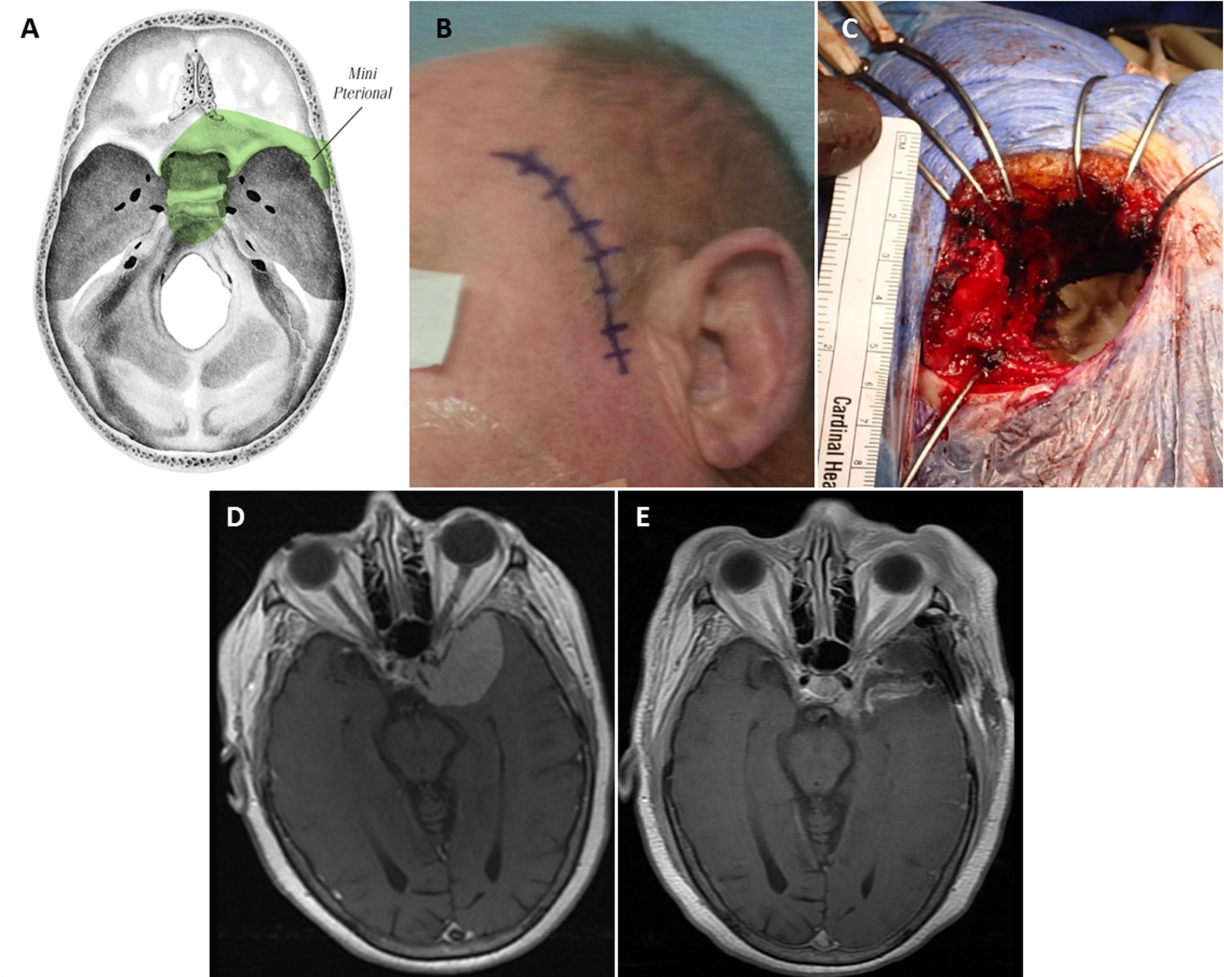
Miniature Pterional Keyhole Approach The skull base regions accessible through this approach (A). Planned incision (B) and craniotomy (C), which is approximately 5.5 cm in largest diameter. Preoperative post-contrast axial T1 MRI shows complex left sphenoid wing and complex skull base meningioma with cavernous sinus invasion (D). Postoperative axial MRI (E).
